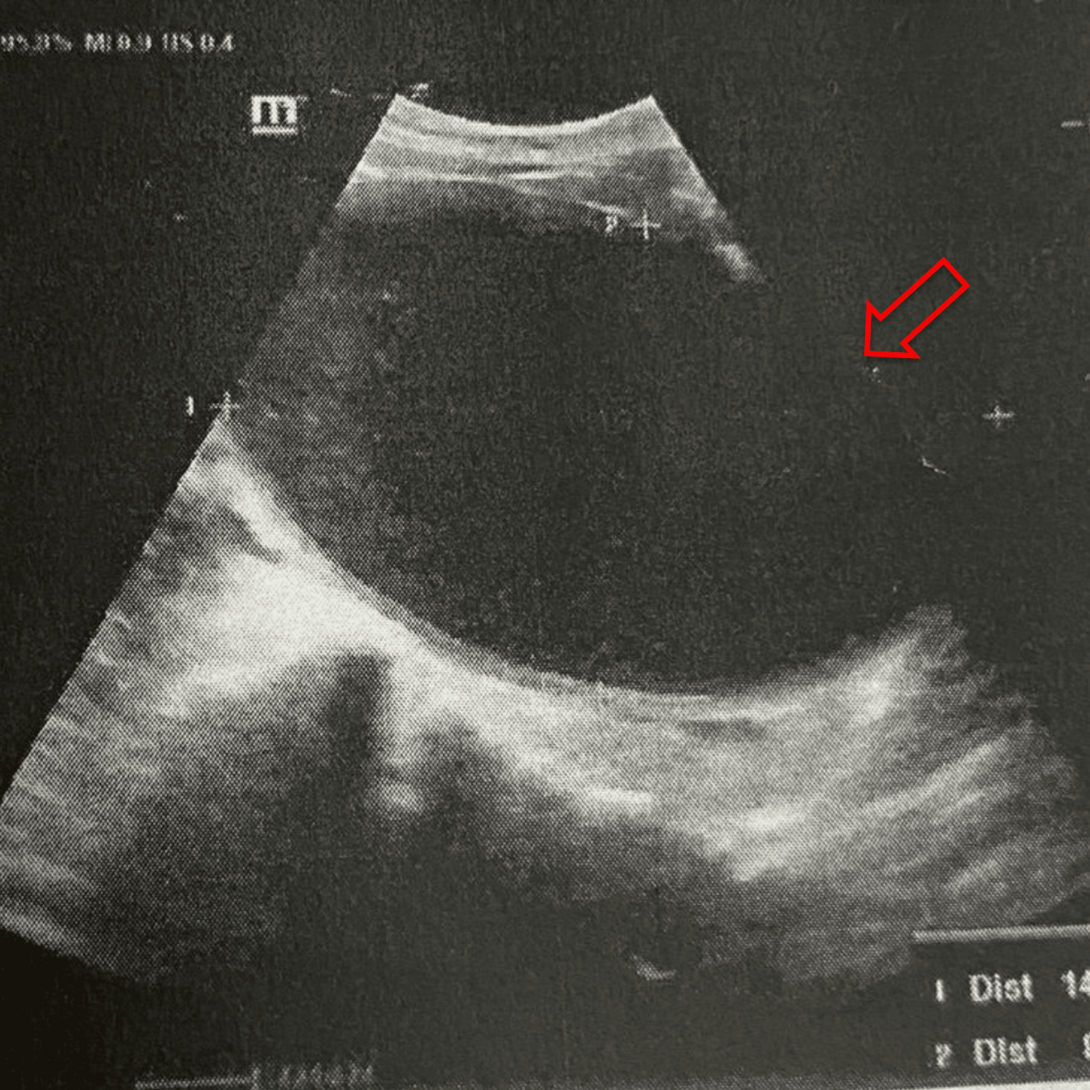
Transabdominal ultrasound shows an anechoic cystic mass at the right adnexa (red arrow).
